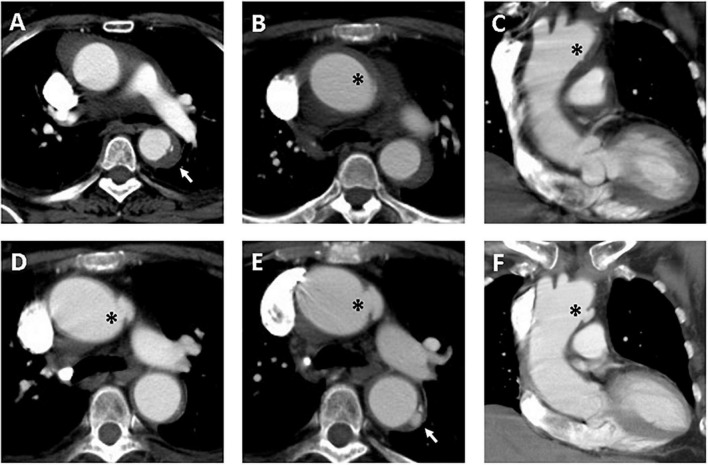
Serial CT scans showed progression of PAU and ULP in a 69-year-old woman with TAIMH. Contrast-enhanced CT scan showed TAIMH with PAU (arrow) in the proximal descending aorta (A) and ULP (asterisk) in the proximal aortic arch (B,C). Three months later, the follow-up CT scan showed enlarged ULP (D, asterisk). The PAU (arrow) in the proximal descending aorta and ULP (asterisk) in the proximal aortic arch were slowly progressed in overall size on 14-month follow-up CT scan (E,F). The patient received medical treatment alone and was still alive.
